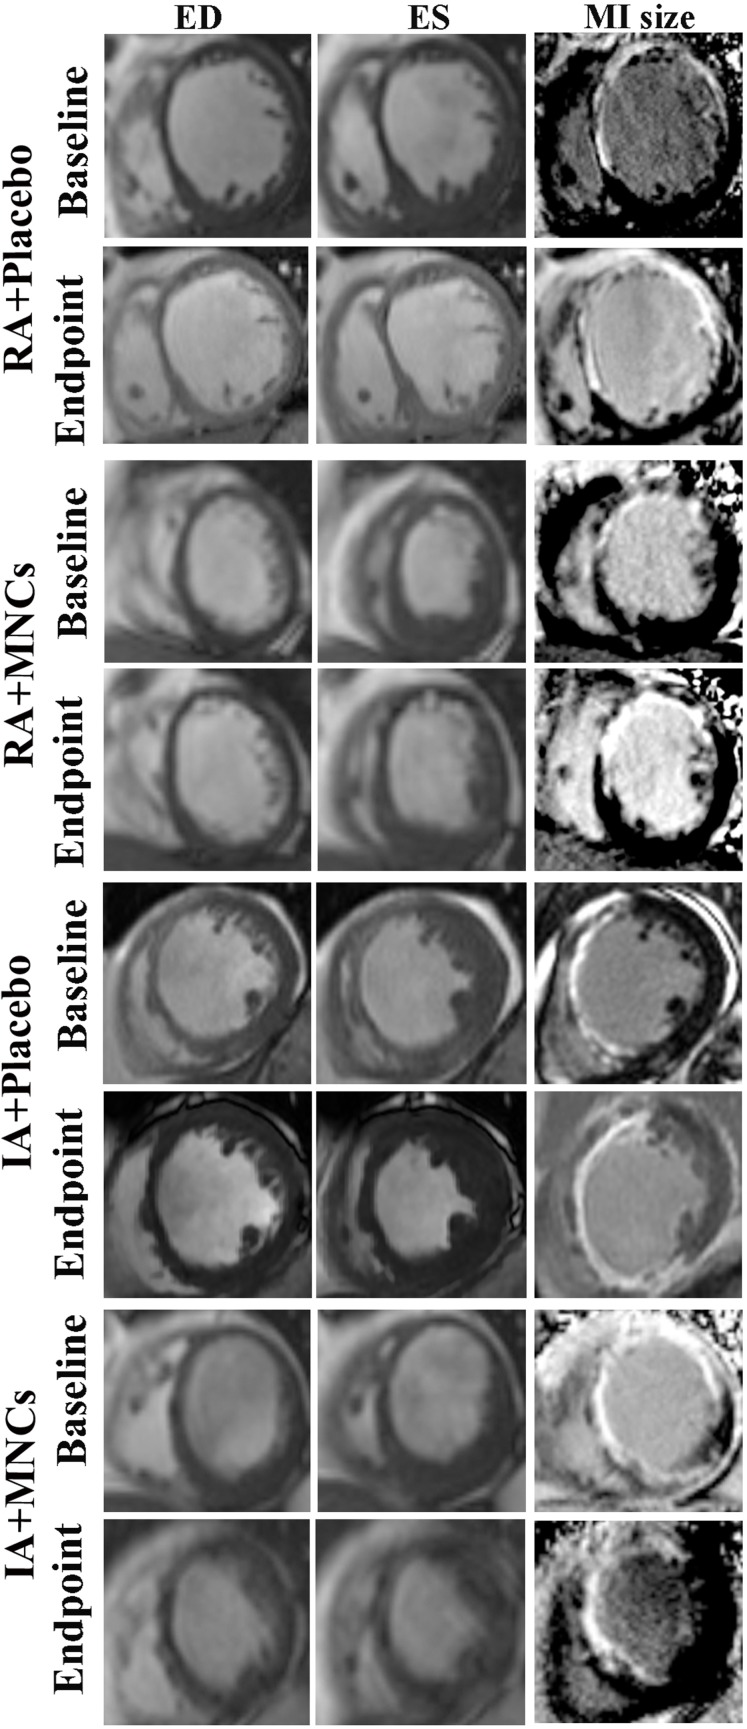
Representative MRI images of cardiac left ventricle in myocardial infarct (MI) patients treated with intensive (IA) or regular atorvastatin (RA) in combination with or without transplantation of mononuclear cells (MNCs). MRI was conducted at short axis in the end-diastolic (ED) or end-systolic (ES) phase.
